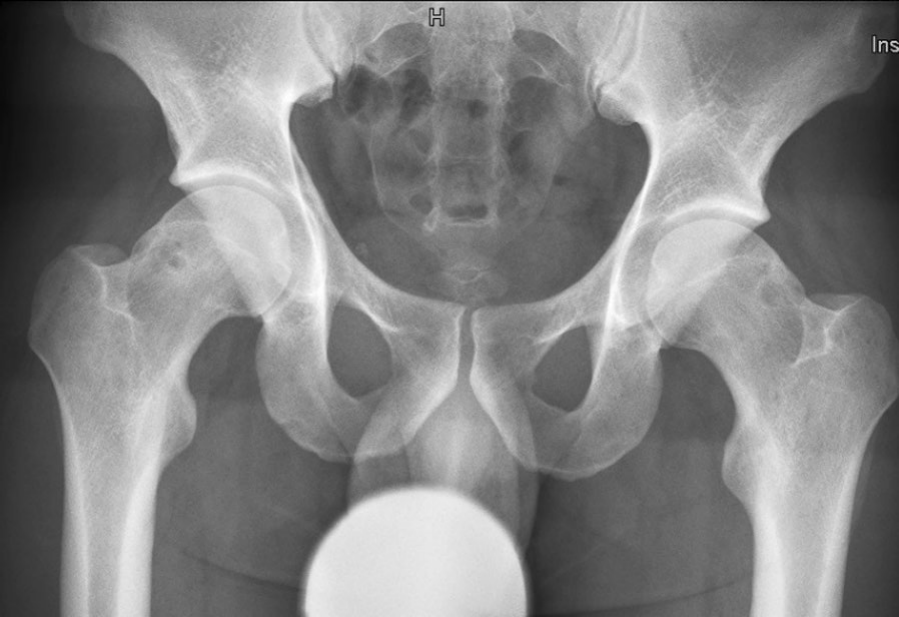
Routine preoperative radiograph showing a bilateral CAM deformity
